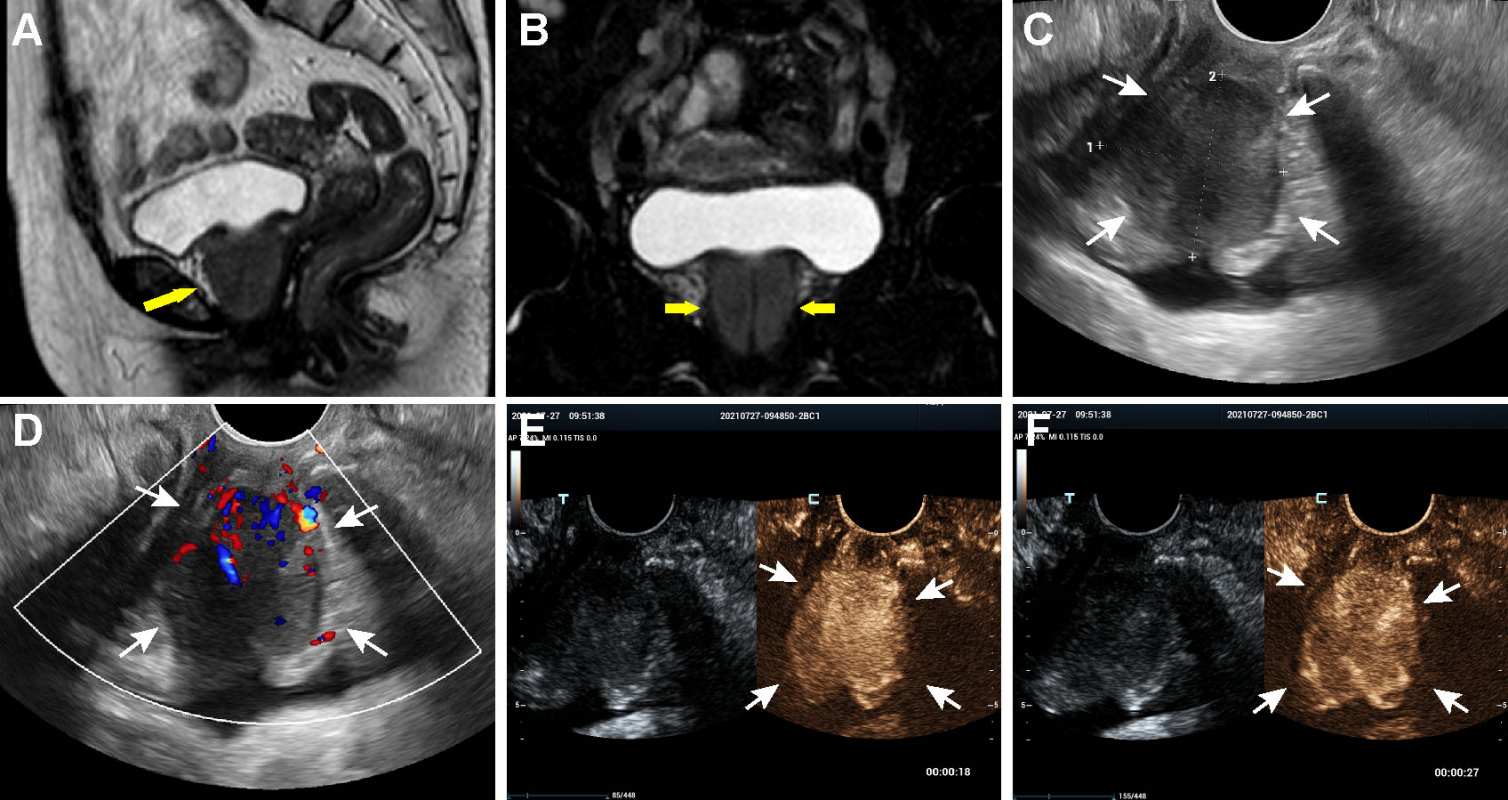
MRI, US and CEUS findings of the periurethral mass on admission. (A, B) Coronal and sagittal MRI of the pelvis, showing a well-defined mass around the urethra (arrows). (C, D) Transvaginal ultrasonography scan revealed a heterogeneously low echoic mass encasing urethra and color Doppler imaging indicated abundant blood flow signals in the lesion. (E) CEUS image obtained at the 18 sec after contrast injection indicating an overall heterogeneously high enhancement of the mass in the arterial phase. (F) CEUS image obtained at the 27 sec showed quick wash-out of the contrast in the lesion.
